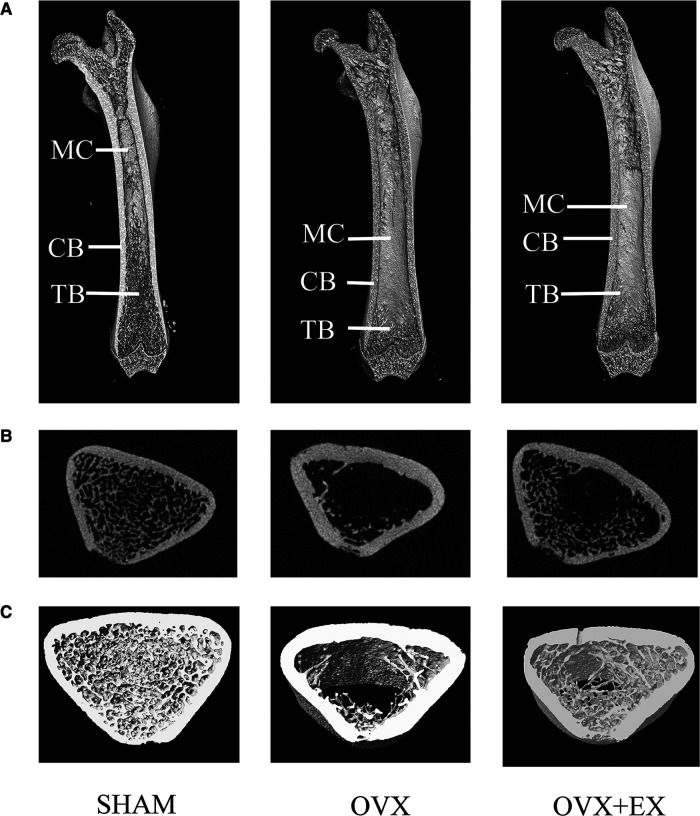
Two-dimensional structure of the longitudinal and transverse sections of the femur and the three-dimensional (3D) reconstruction of cancellous bone of the distal femur in the region of interest: (A) the longitudinal section of the femur; (B) the transverse section of the distal femur; and (C) 3D reconstruction of bone. (Representative micro-computed tomography pictures are shown here). MC, Marrow Cavity; CB, cortical bone; TB, trabecular bone.
